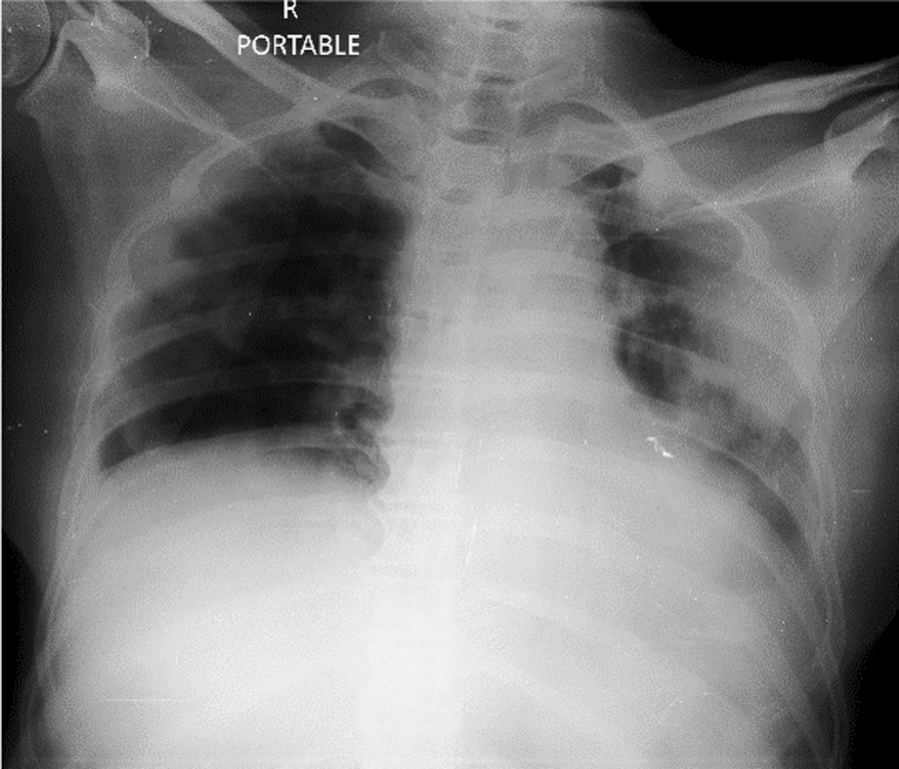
Chest X-ray showing bronchopneumonia in left lung predominantly in the mid-zone
